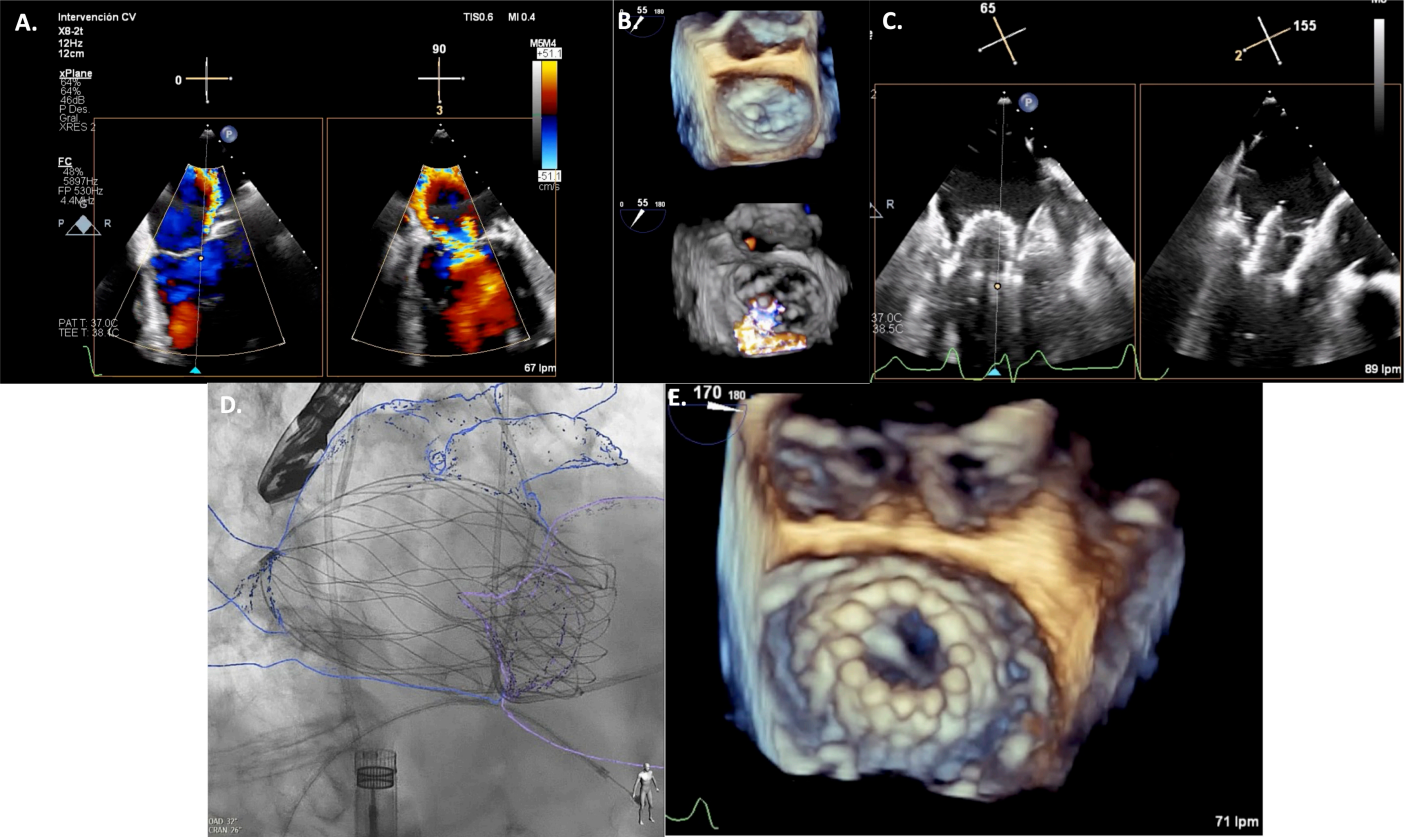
AltaValve implantation procedure. Transoesophageal
